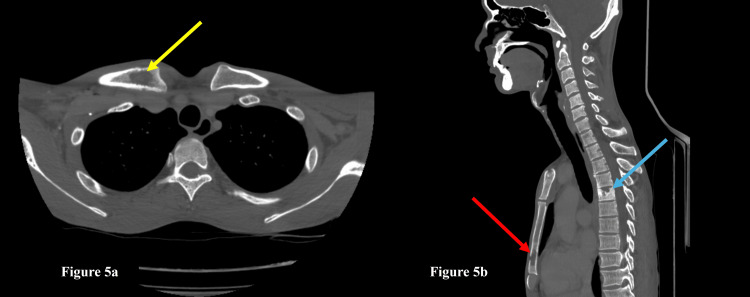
CT chest and abdomen in bone window reconstruction a: The axial section of the CT chest in bone window reconstruction shows a lytic lesion in the right clavicle (yellow arrow); b: The sagittal section of the CT chest in bone window reconstruction shows lytic lesions in the sternum (red arrow) and D5 vertebral body destruction (blue arrow).
